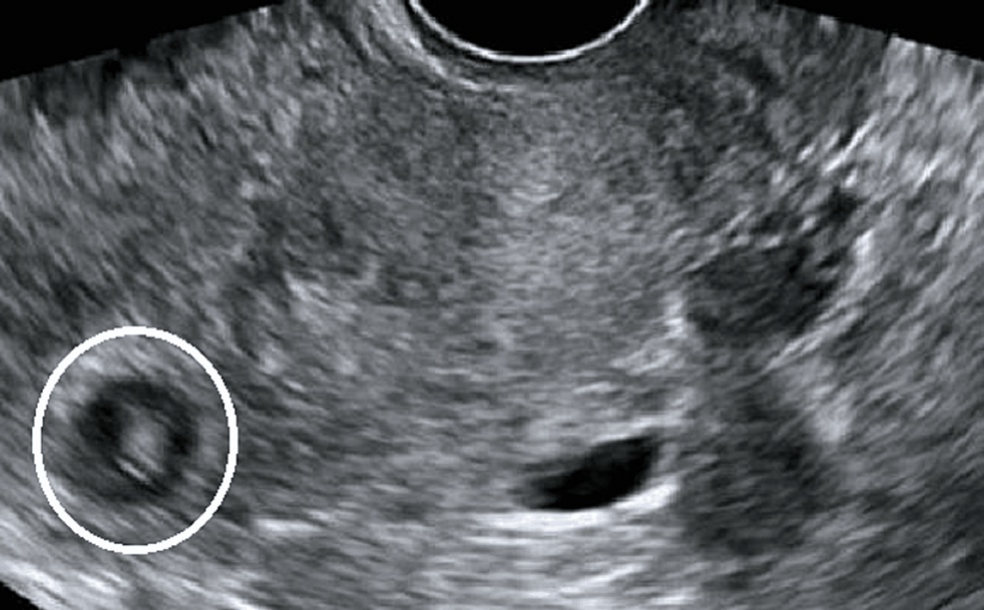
Transvaginal ultrasound shows left adnexal mass (shown by white circle)
